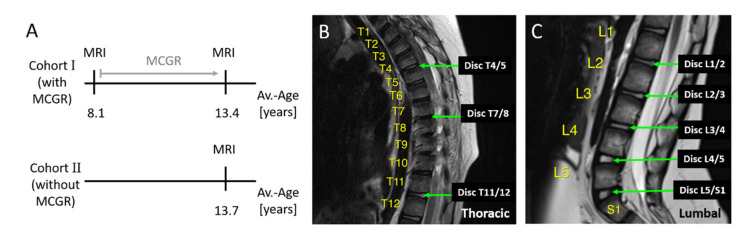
Cohorts and analyzed intervertebral discs. Cohort I had MRI investigations before and after on average 5.1 years of MCGR treatment. Cohort II had an MRI investigation at an average age of 13.7 years thus matching the late MRI exam of cohort I (A). On MRI scans intervertebral discs between thoracic vertebra 4 and 5 (T4/5), 7 and 8 (T7/8) and 11 and 12 (T11/12) were analyzed (B) as well as all intervertebral discs in the lumbar region (C).
